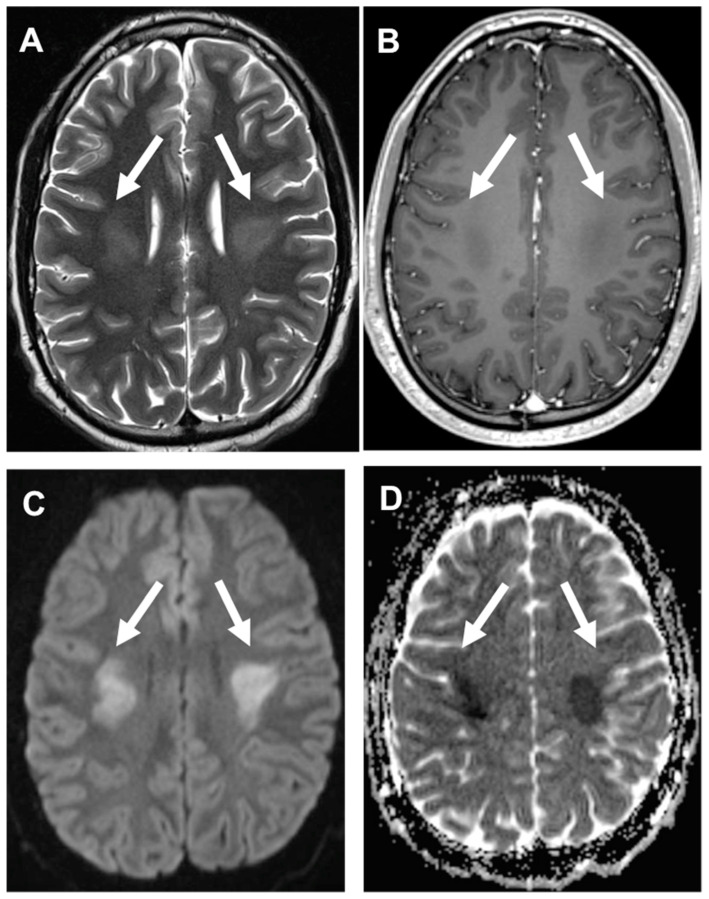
Acute Methotrexate-Induced Neurotoxicity during childhood acute lymphoblastic leukemia (ALL) therapy. MRI shows the characteristic pattern of acute MTX toxicity. Bilateral and symmetric white matter lesion areas of the corona radiata are defined by a high signal on T2 w (arrows, A) and no contrast enhancement after gadolinium administration (B) associated with restricted diffusion on DWI (C) and ADC map (D).
